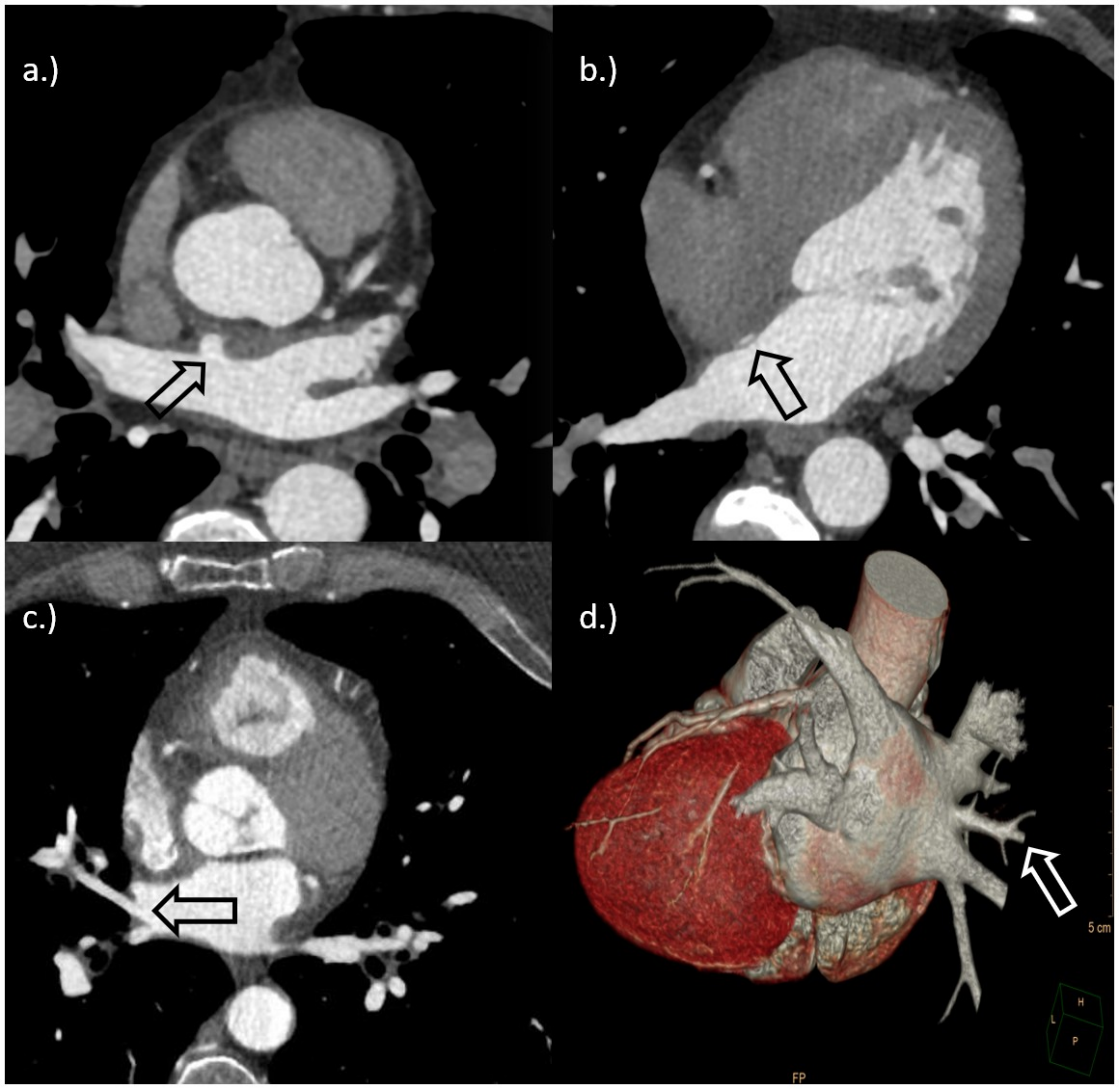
a.) Cardiac-CT of a 55 years old female patient with AF. The arrow shows a single left atrial diverticulum in the anterosuperior wall. b.) Cardiac-CT of a 54 years old female patient with AF. The arrow shows a left-sided septal pouch. c.) + d.) Cardiac-CT and three-dimensional volume rendering of the heart of a 44 years old male patient with AF. The arrows (white and black) show an accessory pulmonary vein merging into the left atrium from the right.
